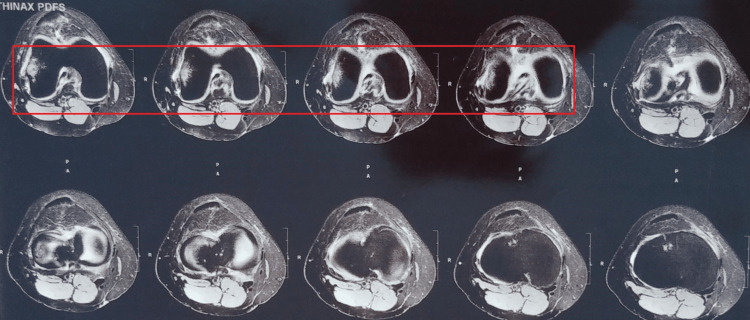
MRI of partial tears of popliteus tendon and anterior horn of the lateral meniscus.
